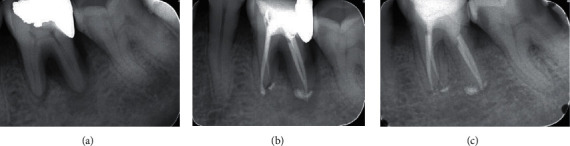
Large size lesion in initial treatment endoperio lesion. A: diagnostic X-ray; B: final obturation; C: 12 month follow-up.
